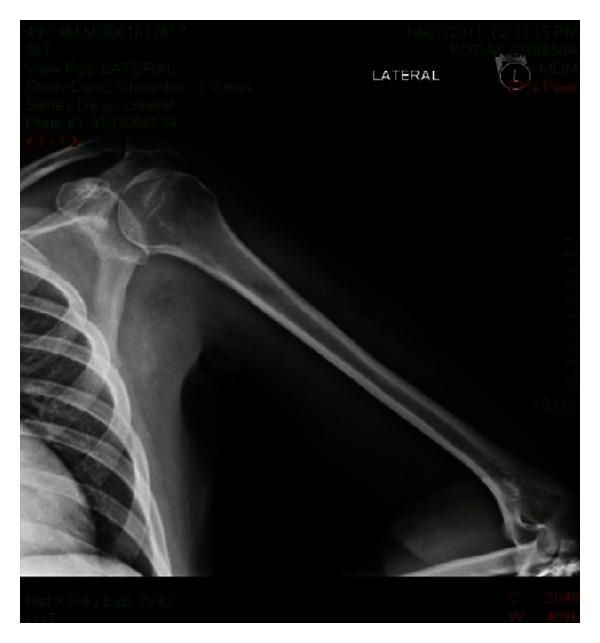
Left shoulder AP X-ray. No subcutaneous gas. Soft tissue swelling.
